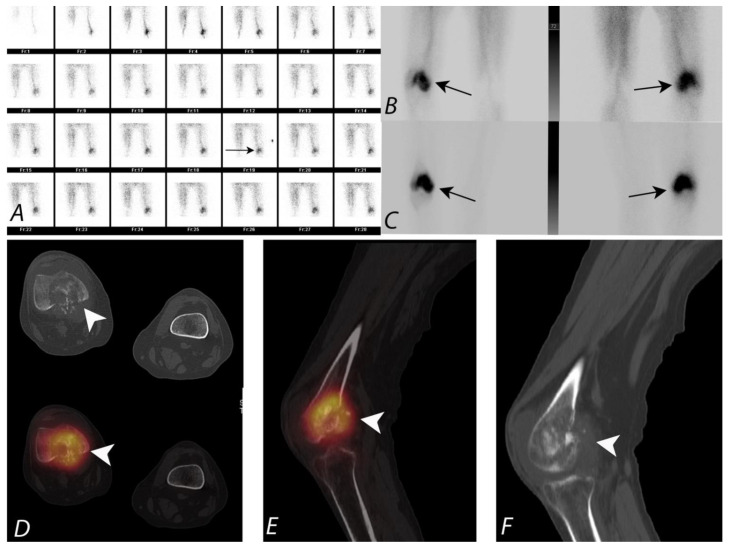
Twenty-year-old male patient presenting with right knee pain. Plain radiograph demonstrated a lesion suspicious for osteosarcoma involving the distal femoral shaft (Not shown). Patient underwent three phase ^99m^Tc MDP bone scan as a part of staging. Blood flow images (A) in posterior projection demonstrated asymmetrically increased blood flow to the right distal femoral region (black arrow). Blood pool and delayed images (B,C) also demonstrated increased pool activity and delayed accumulation of activity, correlating with the region of increased vascularity noted on blood flow images (black arrows). Axial CT and fused SPECT/CT (D), sagittal fused SPECT/CT (E) and sagittal CT images (F) images localize the activity to right distal femoral condyle predominantly lytic lesion associated with osteoid matrix. There is posterior cortical destruction, associated with soft tissue extension of the tumor posteriorly, which also demonstrates activity within (white arrowheads). Small knee joint effusion is also seen.
